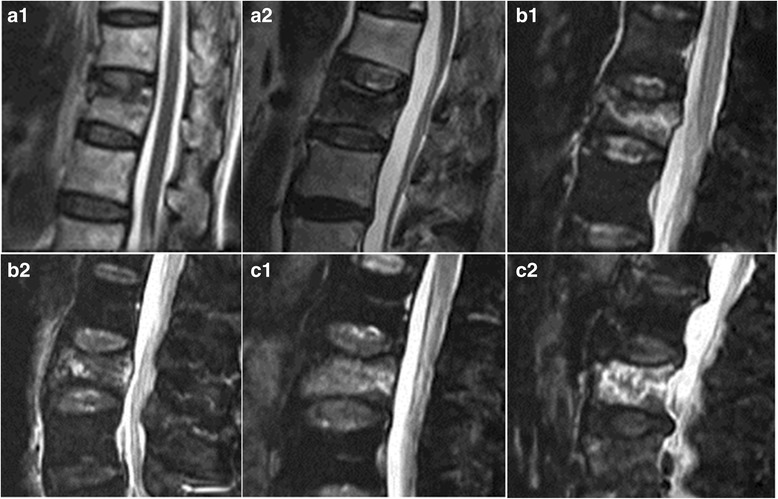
According to the degree and ranges of bone marrow edema on the MRI sagittal view, the patients were divided into mild edema(a1、a2), moderate edema(b1、b2), and severe edema(c1、c2)
